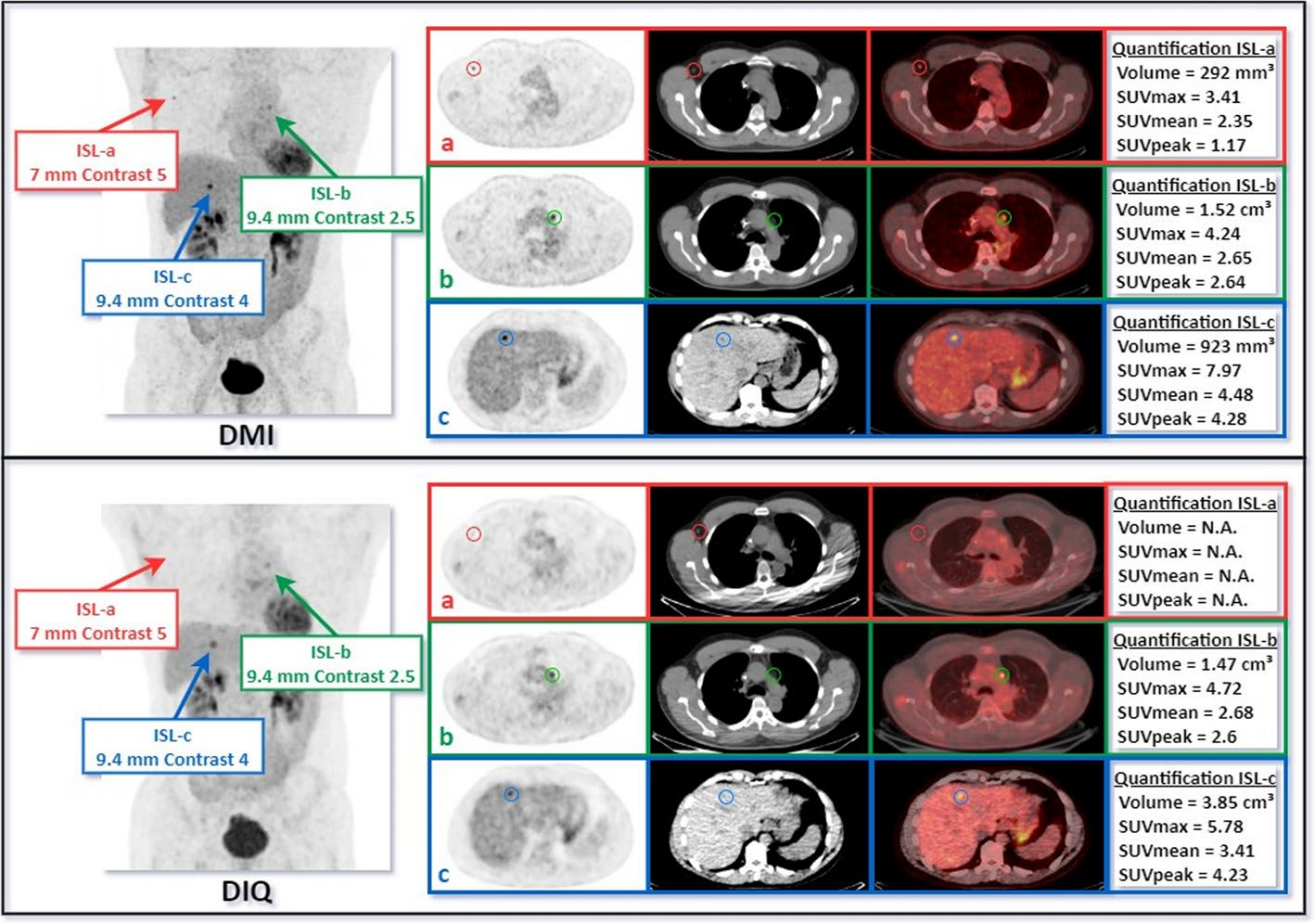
Example of ISL into two consecutive examinations of the same patient. The first examination is displayed at the top (DMI) and the second at the bottom (DIQ). Three synthetic lesions were inserted in three anatomical areas: in an axillary lymph node (a), a lesion 7 mm in diameter and a contrast of 5; in the mediastinum (b), a lesion 9.4 mm in diameter and a contrast of 2.5; in the liver (c), a lesion 9.4 mm in diameter and a contrast of 4. When reviewing the images, the physicians reported three targets on the DMI versus two on the DIQ in their consensus. For each PET/CT scanner we have from left to right: Maximum Intensity Projection (MIP), PET, CT and fused images displayed. Semi-quantitative metrics are reported in box next to axial PET, CT and fused images
